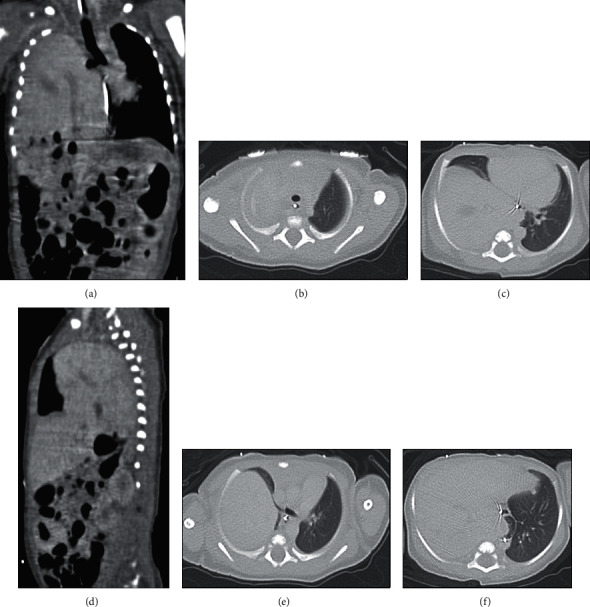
Computed tomography scans one day after birth. (a) Coronal view of the liver in the right hemithorax extending to the apex with leftward mediastinal shift. (b) Axial images depicting the apex of the lungs, with the right lung apex completely compressed by the liver. (c) Axial view showing the leftward mediastinal shift and the extent of aeration of the right middle lobe. (d) Sagittal view of right lung at the level of greatest aeration. (e) Axial view depicting the right mainstem bronchus and cardiothymic shift. (f) Axial view suggesting possible aplasia of the right lower lobe.
